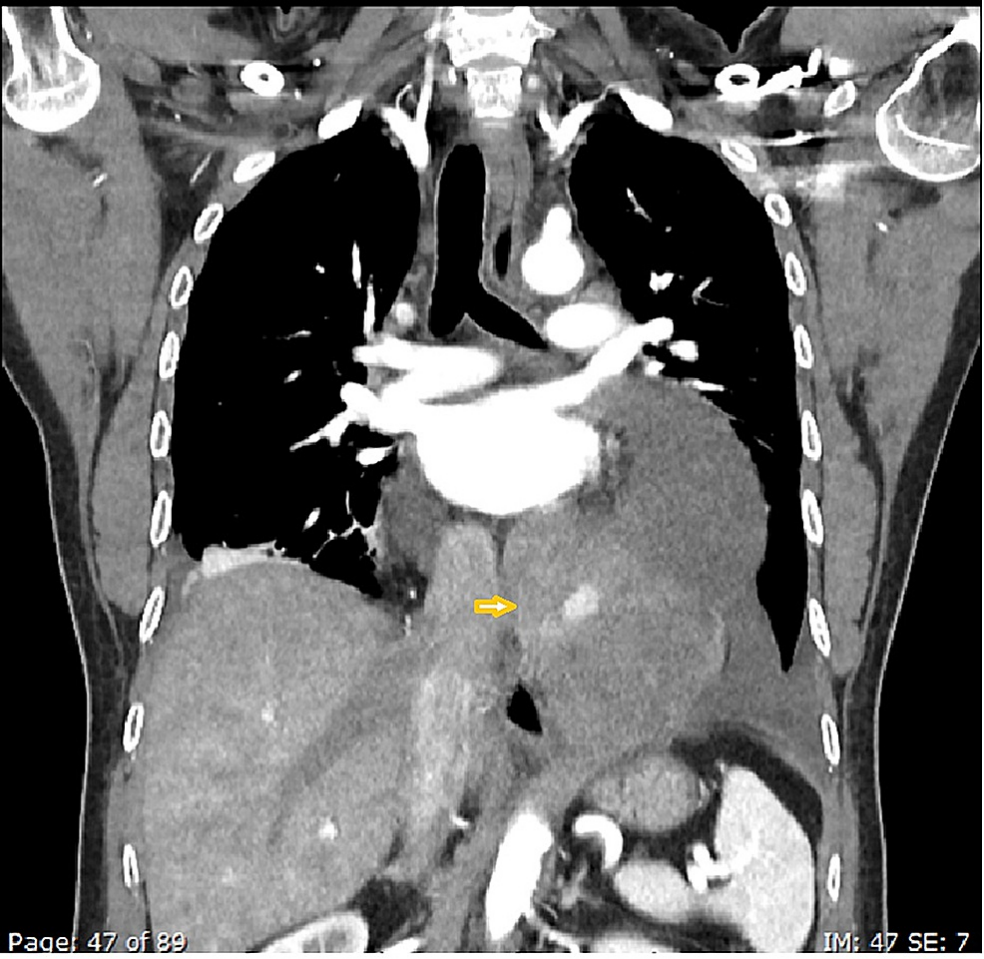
Coronal contrast CT (arterial phase) images showing a large, heterogeneously enhancing pericardial mass (arrowhead)
